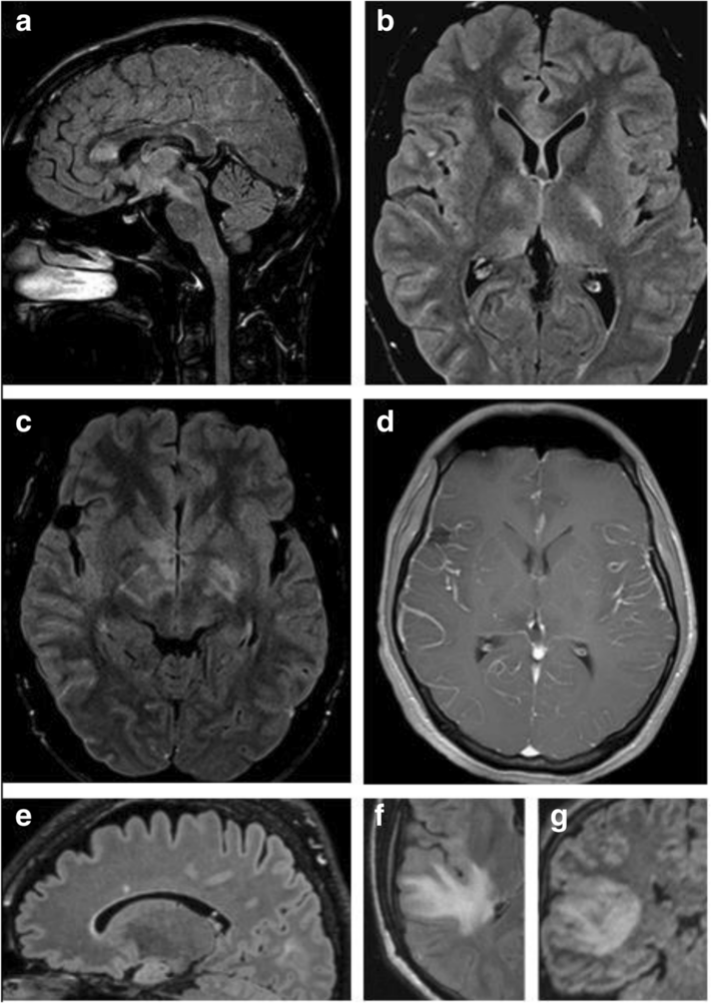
Examples of brain lesions detected by MRI. a Sagittal FLAIR image showing callosal lesions as well as lesions extending from the diencephalon to the pons (see case 8 in part 3 of this article series [31] for details). b Axial FLAIR MRI demonstrating lesions in the basal ganglia, juxtacortically on the right side, und in the genu corporis callosi in the same patient. c Axial FLAIR image at the diencephalic level revealing periependymal lesions (in addition to basal ganglia lesions). d Axial T1-weighted image with Gd demonstrating leptomeningeal enhancement (see case 8 in part 3 [31]). E: Sagittal MRI showing a callosal lesion (see case 10 in the Appendix for details). f, g Axial T2-weighted (f) and coronal FLAIR (g) images showing large, confluent T2 hyperintense lesions in the right temporal lobe (see case 7 in part 3 [31])
